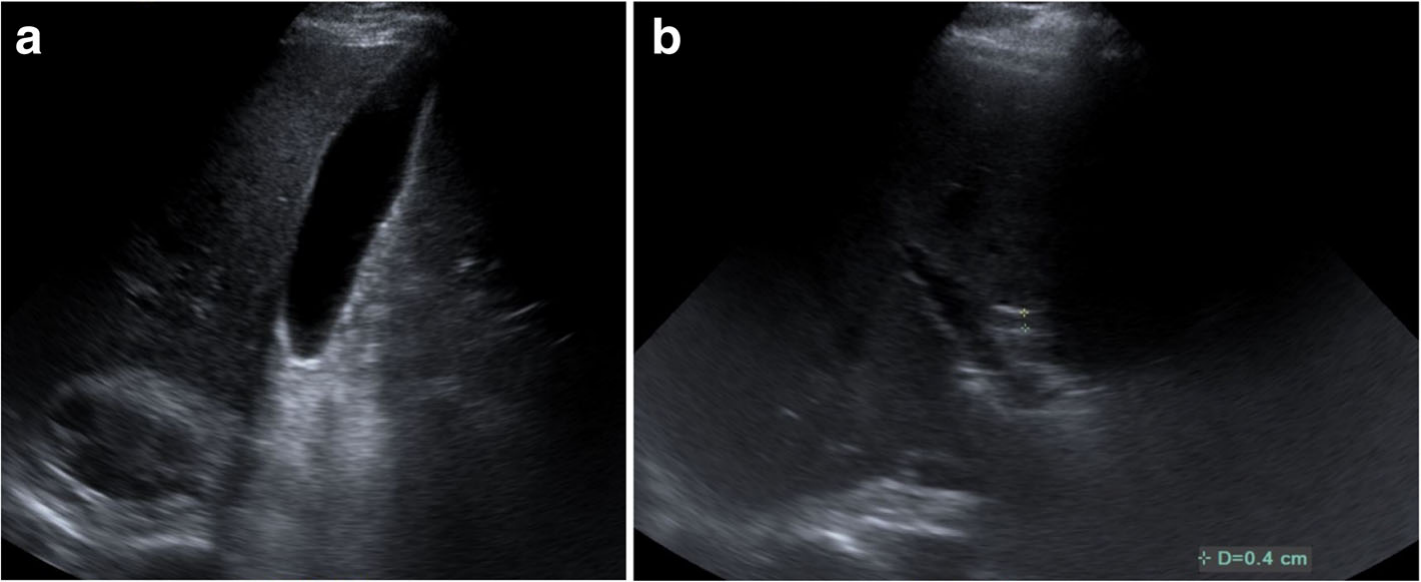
Abdominal ultrasound images. a Gallbladder without any stones. b Normal caliber common bile duct ≤ 7 mm demonstrated
